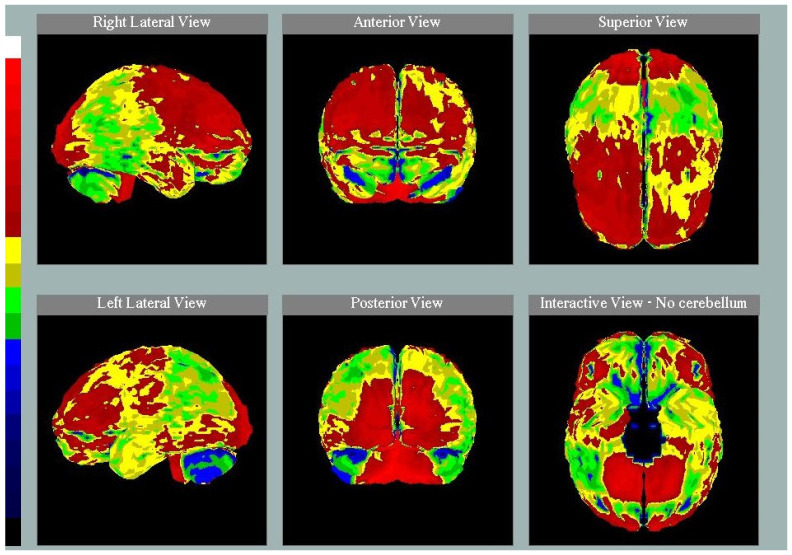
Single-photon emission computed tomography (SPECT) of the patient with the use of Stereotactic Surface Projection (SSP) method and cerebellum as reference standard, showing bilateral hypoperfusion of the parietal and posterior temporal lobes. The color scale on the left side of the image represents perfusion in the brain cortex compared with perfusion of cerebellum in descending order from the top (red color, corresponding to normal perfusion) to the bottom (blue color, corresponding to severely reduced perfusion).
